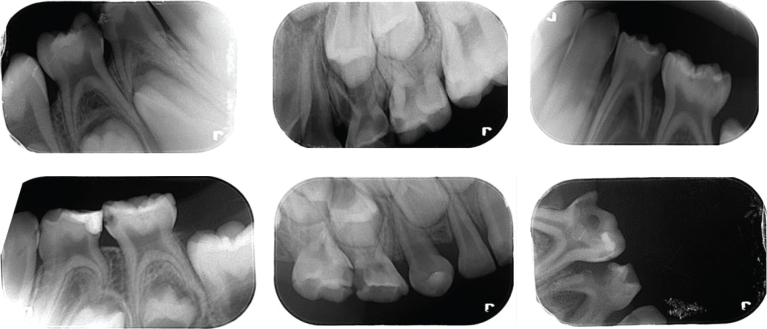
Some of the collected radiographic images.
